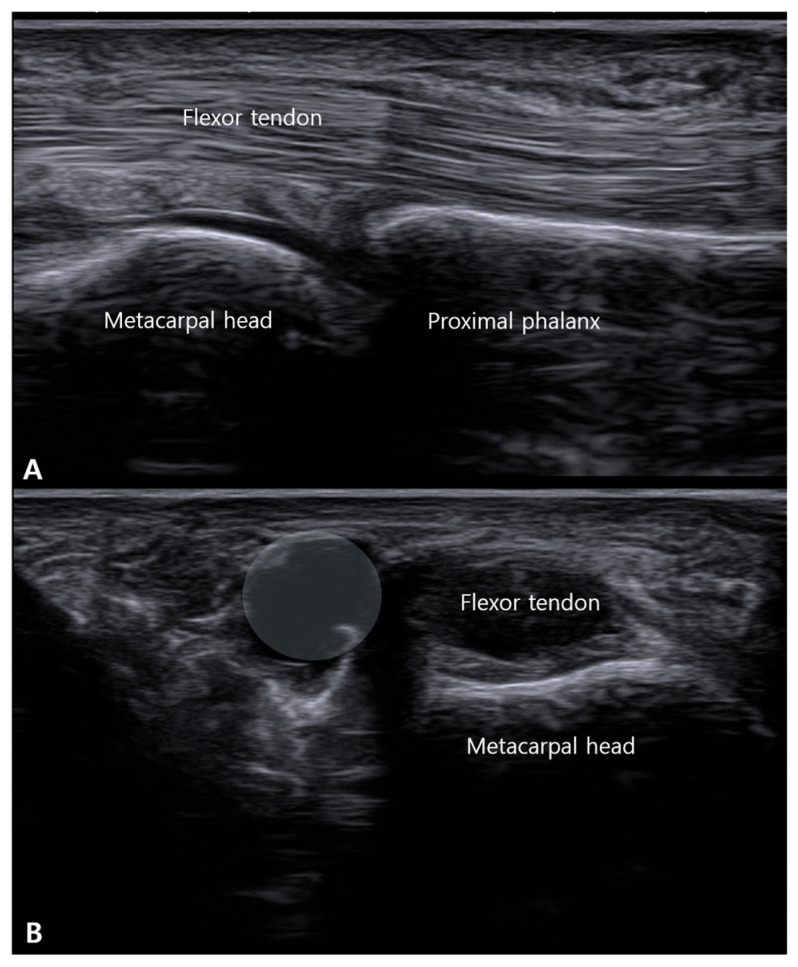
The biplanar ultrasound assessment for detecting an A1 pulley ganglion. (A) Longitudinal sonographic view over the A1 pulley region showing no apparent abnormality of the flexor tendons. (B) Corresponding short-axis (transverse) view at the same level reveals a well-defined hypoechoic ganglion cyst (whited shaded region) located on the radial aspect of the pulley. This case underscores the necessity of orthogonal imaging planes to avoid missing laterally situated pathology.
